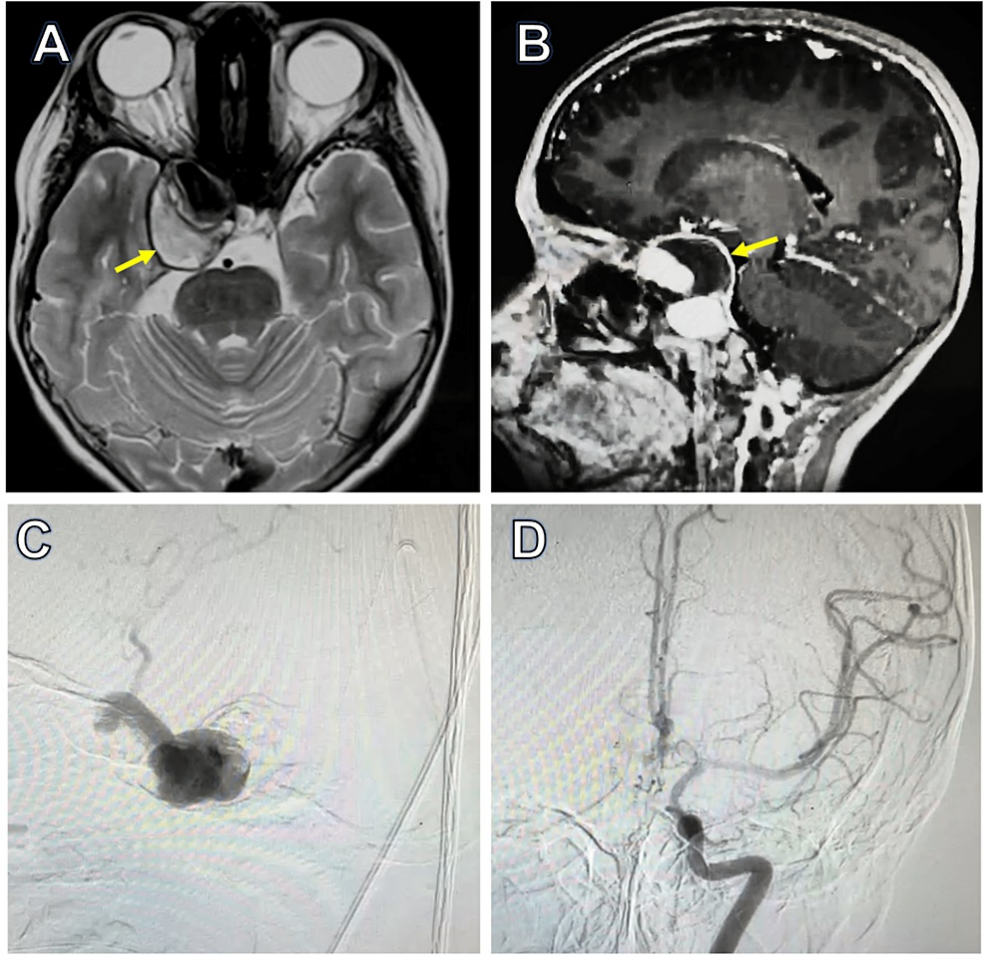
Preoperative scans. MRI axial (A) and sagittal views (B) show an aneurysm with partial thrombosis of 54 mm in diameter in the cavernous segment of the right ICA (arrows). The angiography images (C) and (D) confirmed the presence of the aneurysm with poor collateral circulation. MRI: magnetic resonance imaging, ICA: internal carotid artery
